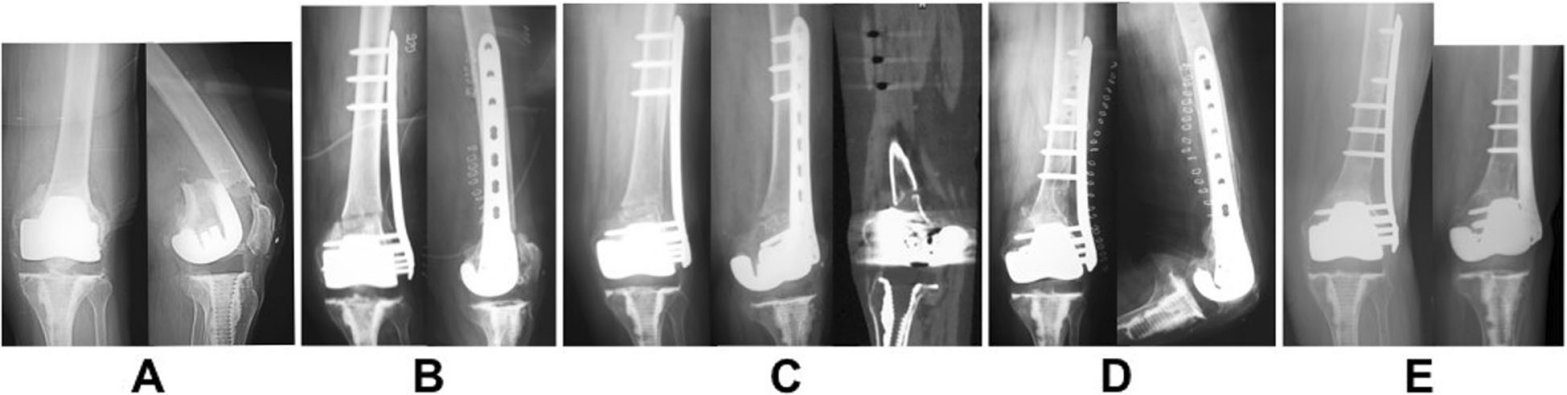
a Radiograph in a 74-year-old woman with total knee arthroplasty. b Postoperative radiograph. c Radiograph and CT showed no callus at 6 months postoperatively. d Screws were inserted near the fracture during re-operation. e Radiograph 1 year after re-operation
